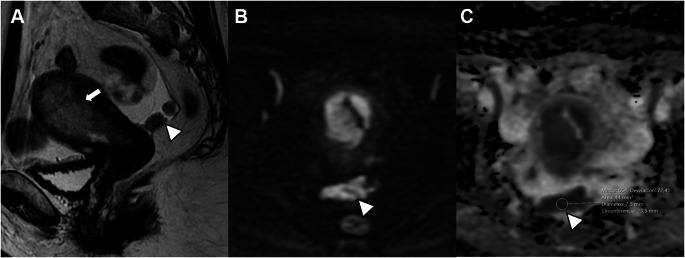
Stage IIIB2 uterine serous carcinoma in a 70-year-old woman. A Sagittal T2-weighted fast spin-echo (FSE) image (TR/TE: 4500/100 ms; slice thickness: 3 mm), B axial diffusion-weighted image (DWI) with b = 1000 s/mm^2^, and C ADC map show a uterine mass (arrow) and peritoneal implants in the Douglas pouch (arrowheads). ADC value in the peritoneal implant is 0.65 × 10^−3^ mm^2^/s
